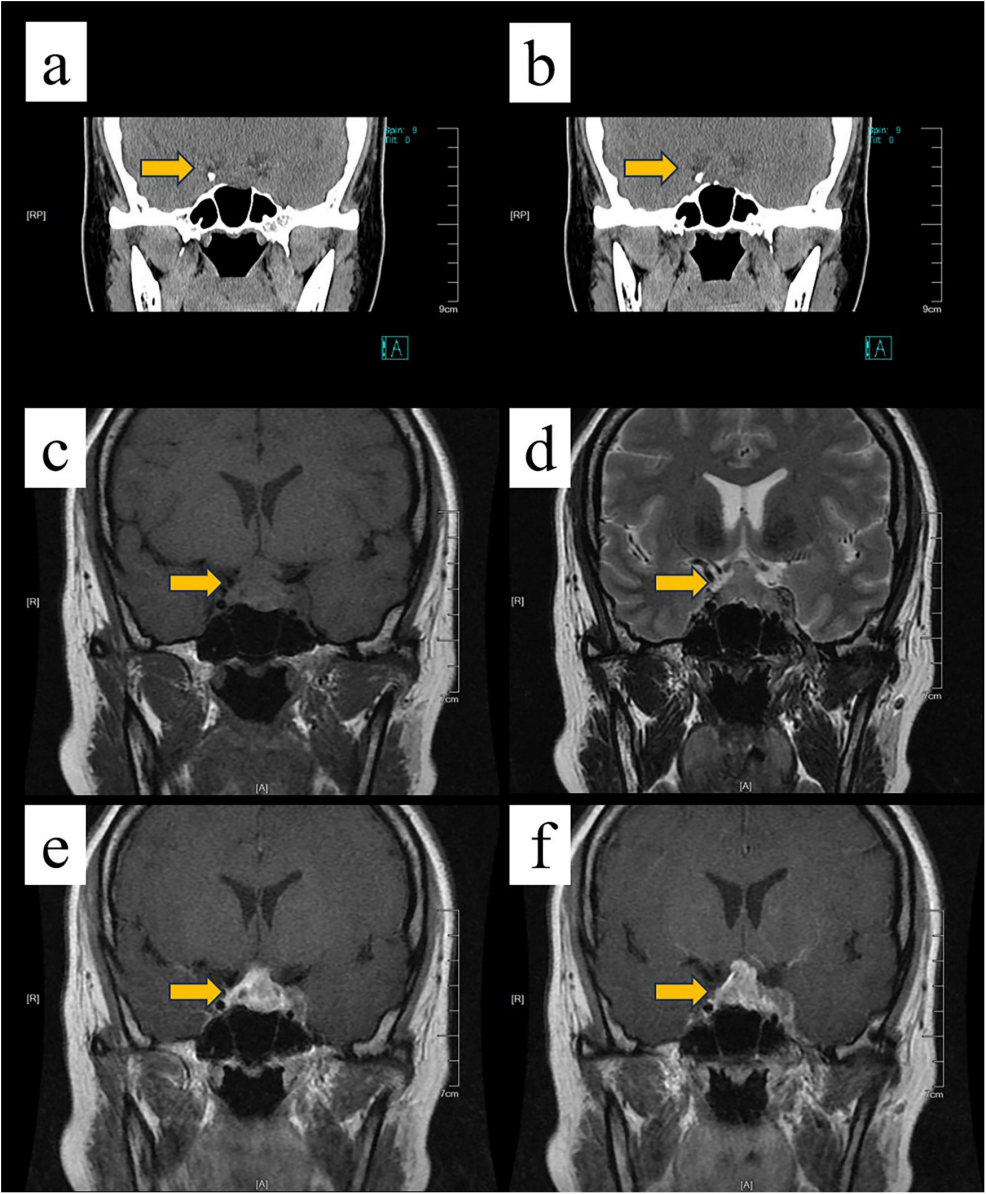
Preoperative neuroimaging findings of the sellar region mass. Cranial computed tomography (CT) scan (a,b) shows a thick soft tissue mass in the sella turcica. Note the absence of calcification within the mass, as well as the compression and resorption of the surrounding bone (arrow). Magnetic resonance imaging (MRI) sequences (c,d). T1-weighted image (c): The right and middle portions of the mass are isointense, while the left portion is slightly hyperintense. T2-weighted image (d): The right and middle portions show an isointense to slightly hyperintense signal, and the left portion is isointense. Contrast-enhanced (e,f): The lesion enhances significantly. The enhancement is heterogeneous in the right and middle portions and mild in the left portion. The mass envelops the pituitary gland and stalk, and the left internal carotid artery appears embedded (arrowhead).
